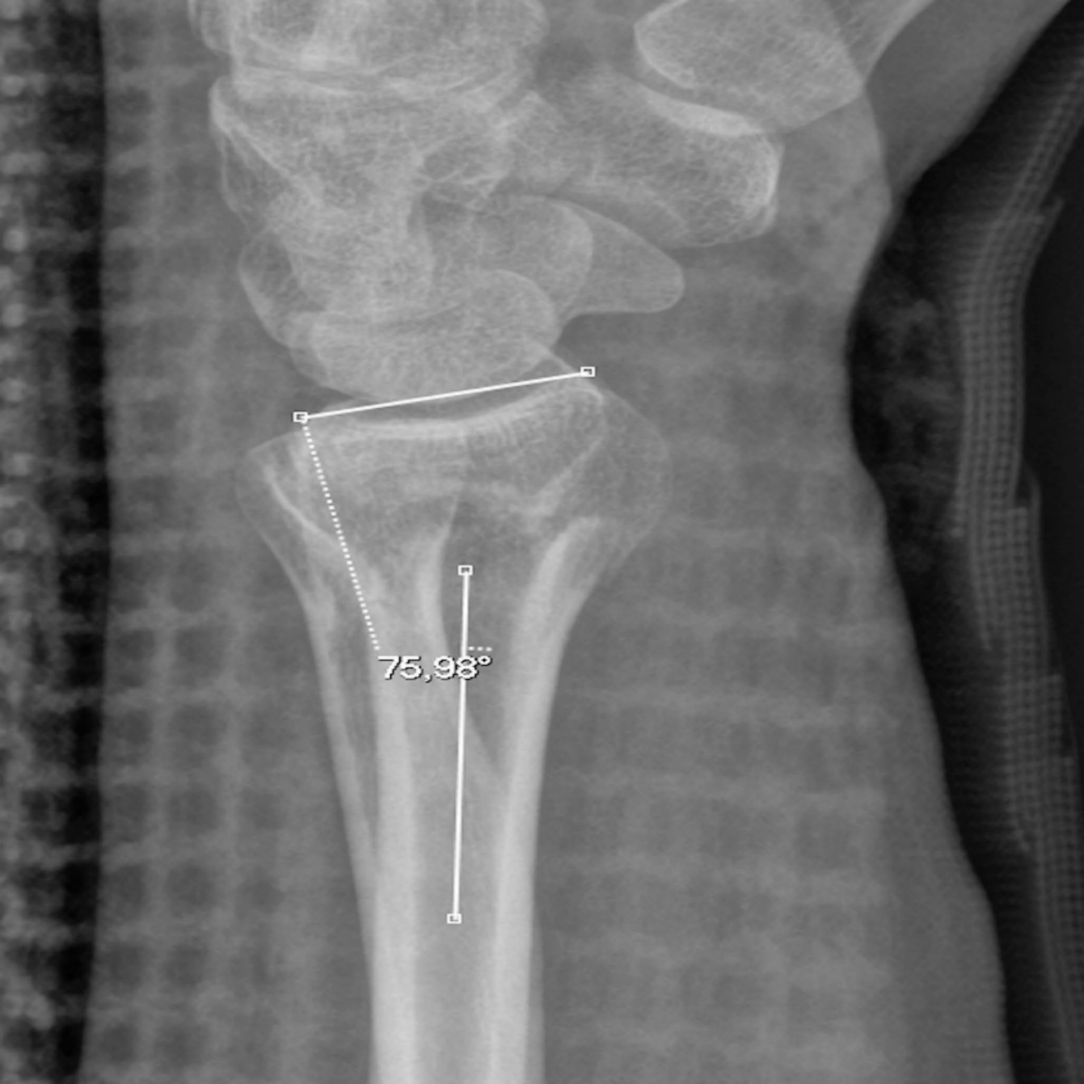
35-year-old male with left sided extraarticular distal radius fracture. Figure 2 shows the true lateral radiograph. Notice the scaphopisocapitate criterium and distal radioulnar overlap, as well as measured dorsal tilt
